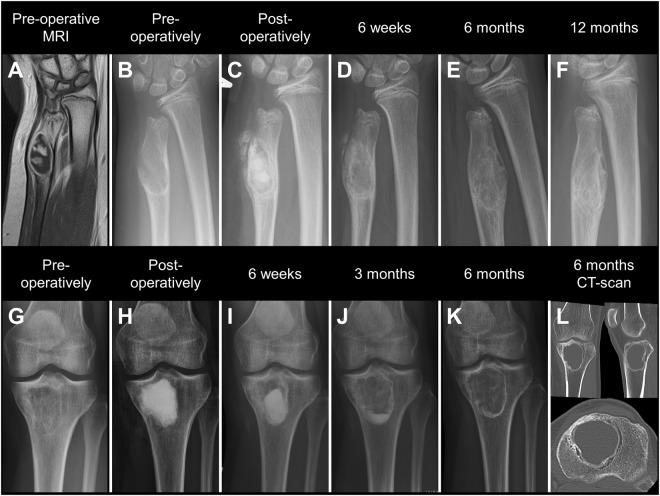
Upper row: Enchondroma of a 12-year old girl treated by curettage and defect-filling using CERAMENT|BONE VOID FILLER. (A,B) Pre-operative MRI and x-ray showing decreased cortical thickness. (C) X-ray taken post-operatively showing near complete defect-filling. (D,E) X-rays taken after 6 weeks, and 6 months showing increasing product resorption and cortical thickening. (F) X-ray taken after 12 months showing normal cortical thickness and signs of cavity-remodeling. Lower row: Large bone infarction of the proximal tibia treated by curettage and defect-filling using CERAMENT|BONE VOID FILLER. (G,H) Pre- and post-operative x-rays showing decreased cavity radiolucency and near complete defect-filling. (I–K) X-rays taken after 6 weeks, and 3 and 6 months showing increasing product resorption and persistent reduced defect-radiolucency. (L) 6-months CT-scan showing a peripheral reactive zone in the defect and a seemingly empty cavity.
